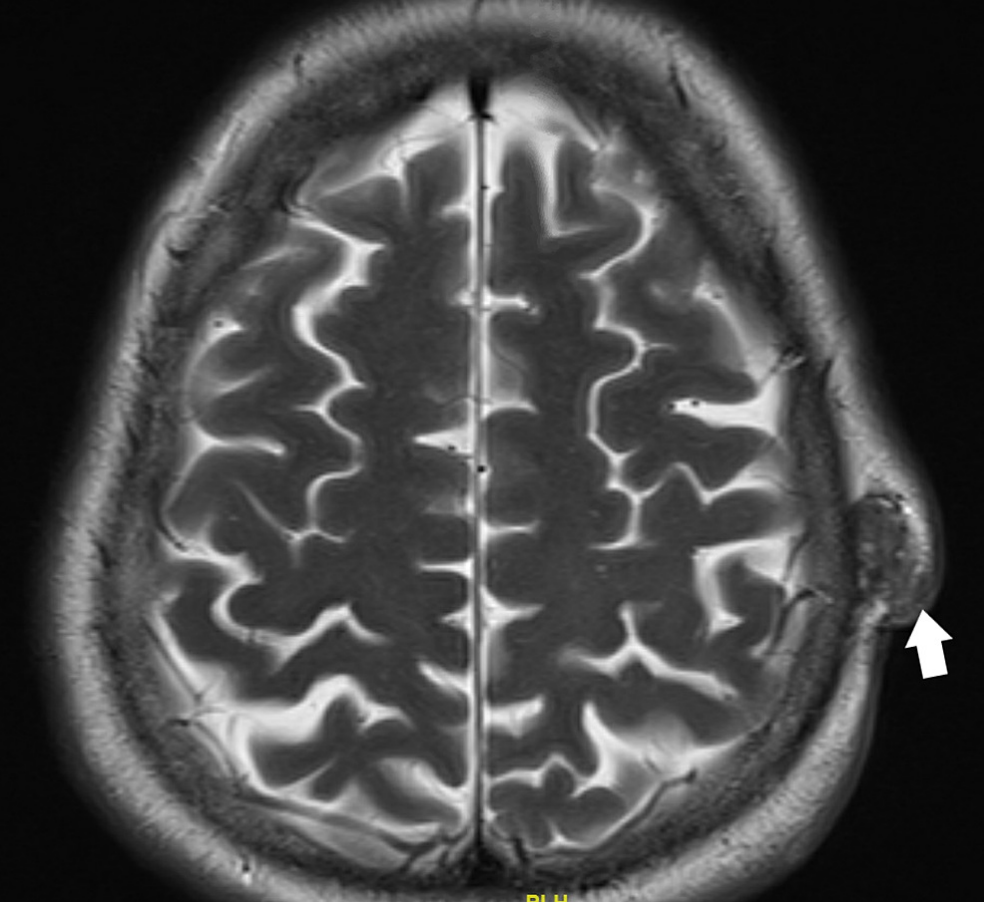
MRI showing the upper lesion (white arrow).
